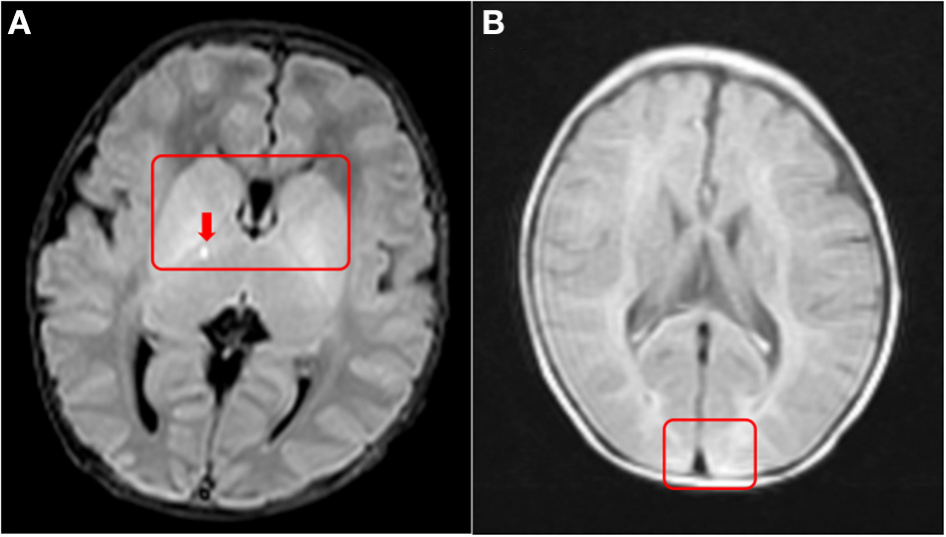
Brain MRI at 10 days and 3 months of age. (A) T2W: Red boxes indicate a high signal of basal ganglia, swelling of bilateral lenticular nucleus; Red arrows indicate an abnormal signal point of posterior branch of right internal capsule. (B) T2W: Red boxes indicate white matter of occipital lobe has high signal, and the boundary of gray and white matter is not clear.
